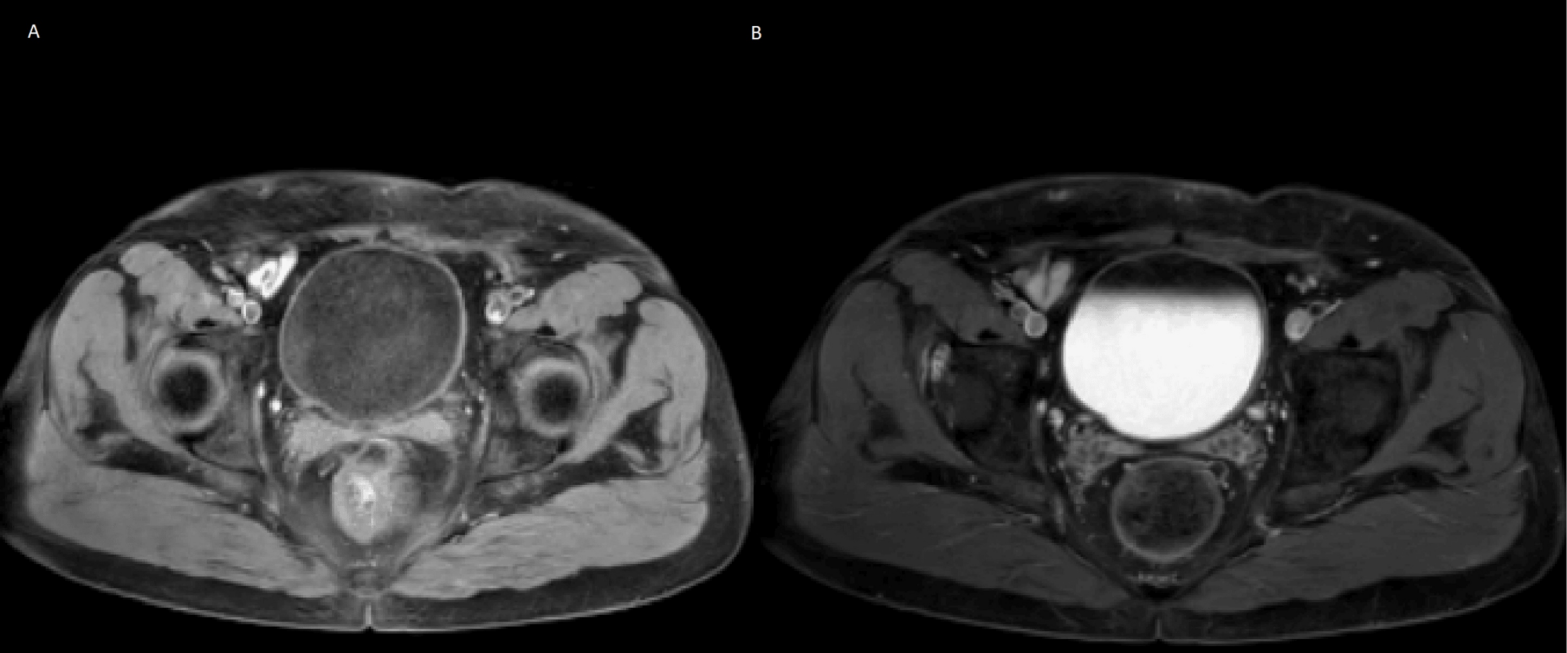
Magnetic resonance imaging of the lower abdomen. A: T1-weighted imaging; B: T1-weighted imaging with contrast enhancement. No remarkable findings were observed.
